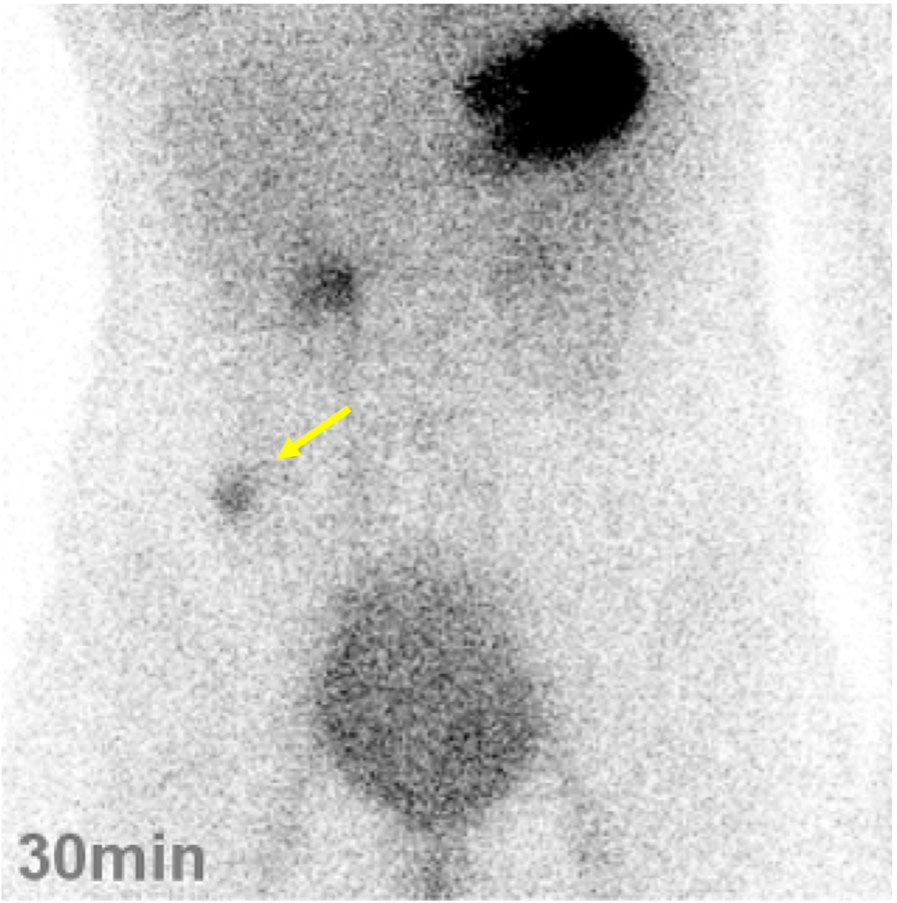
Technetium-99m pertechnetate scintigraphy image showing a hot spot in her lower right abdomen (*arrow*)
